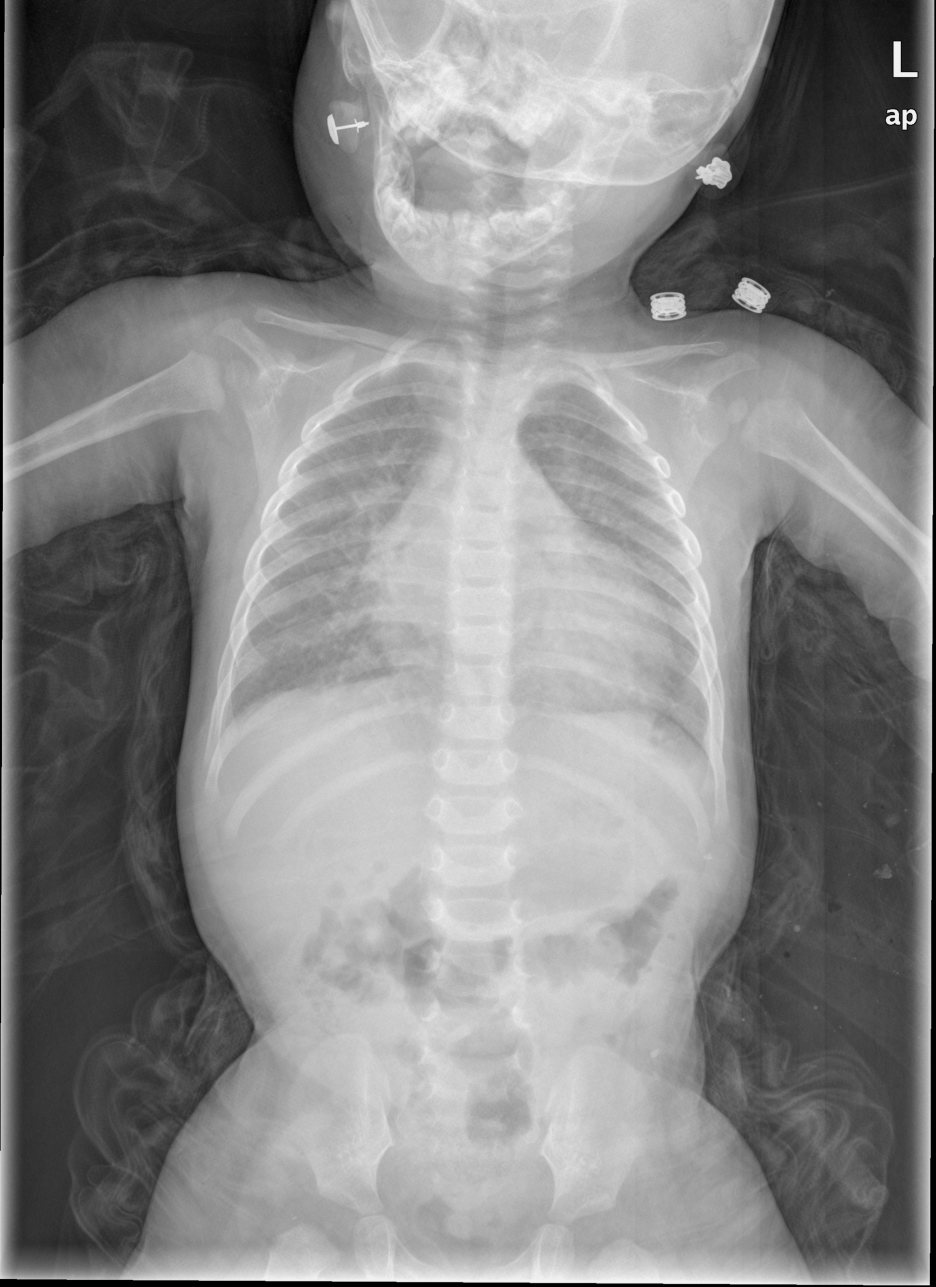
Anteroposterior chest radiograph showing cardiomegaly with increased cardiothoracic ratio and mild pulmonary congestion. These findings are consistent with acute cardiac dysfunction.
